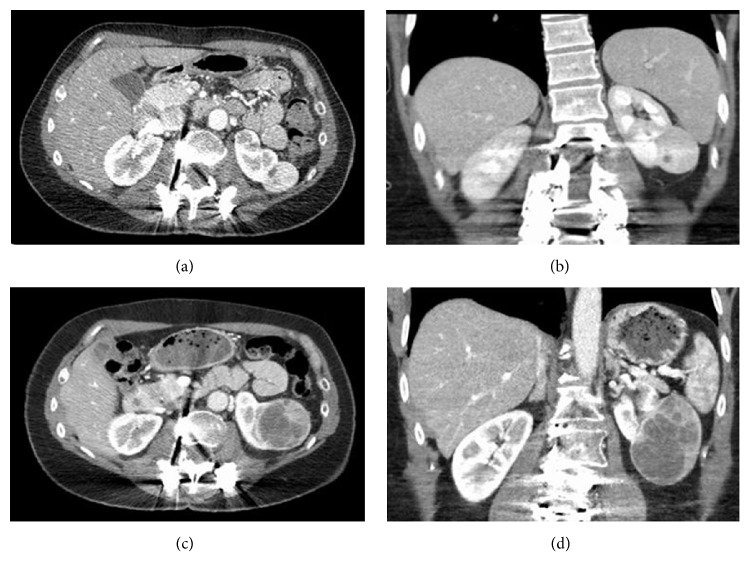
(a)–(d) CT axial and coronal scans depicting an exophytic mass in the lower pole of the left kidney: (a, b) initial presentation showing a well-circumscribed, enhancing mass with two small discrete, low attenuation areas in the inferior portion. (c, d) Enlargement of the renal tumor to almost twice the size from prior examination with compression of calyces, increased heterogeneity, and enhancement at the peripheral and anterior aspects of the mass.
